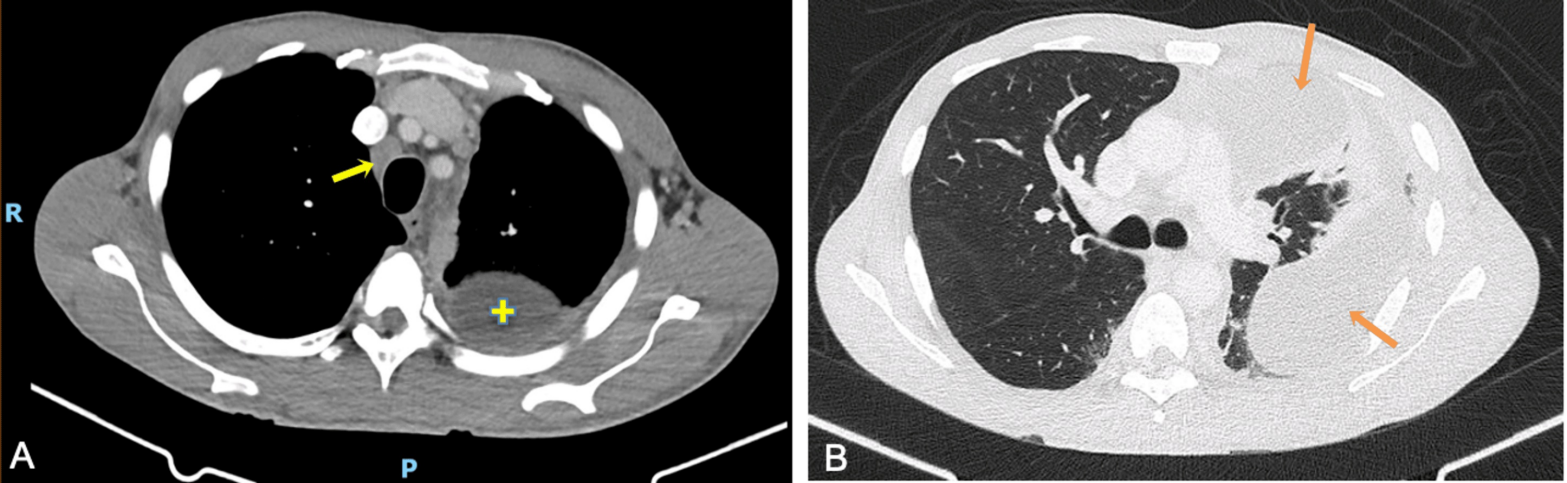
CT scan on hospital admission, transverse plane. Image A shows 4R mediastinal lymphadenopathy (yellow arrow) and an enclosed pleural effusion on the left side (+). Image B, at the level of the carina, highlights the enclosed pleural effusion on the left side (orange arrows).
